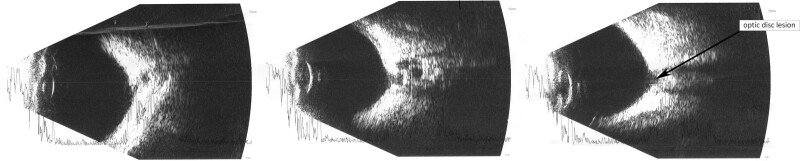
The result of B-scan ultrasonography examination.
